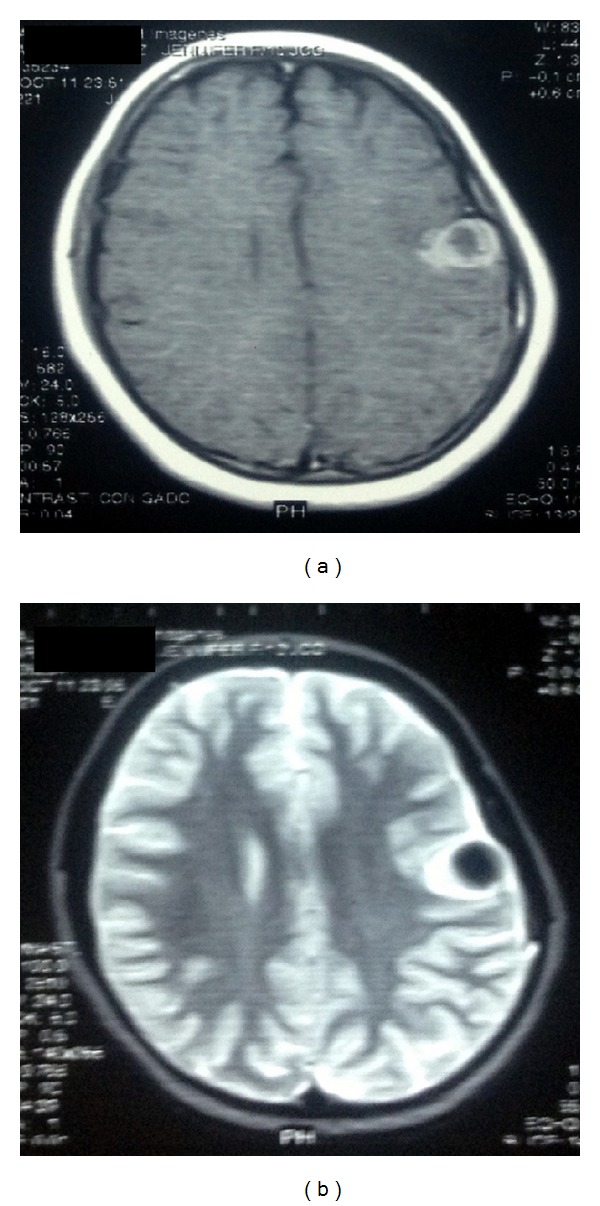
Preoperative MRI: T1-weighted axial image showing an iso-hypointense lesion (a) with peripheral contrast enhancement and a T2-weighted axial hypointense image (b) without mass effect or ventricle involvement.
